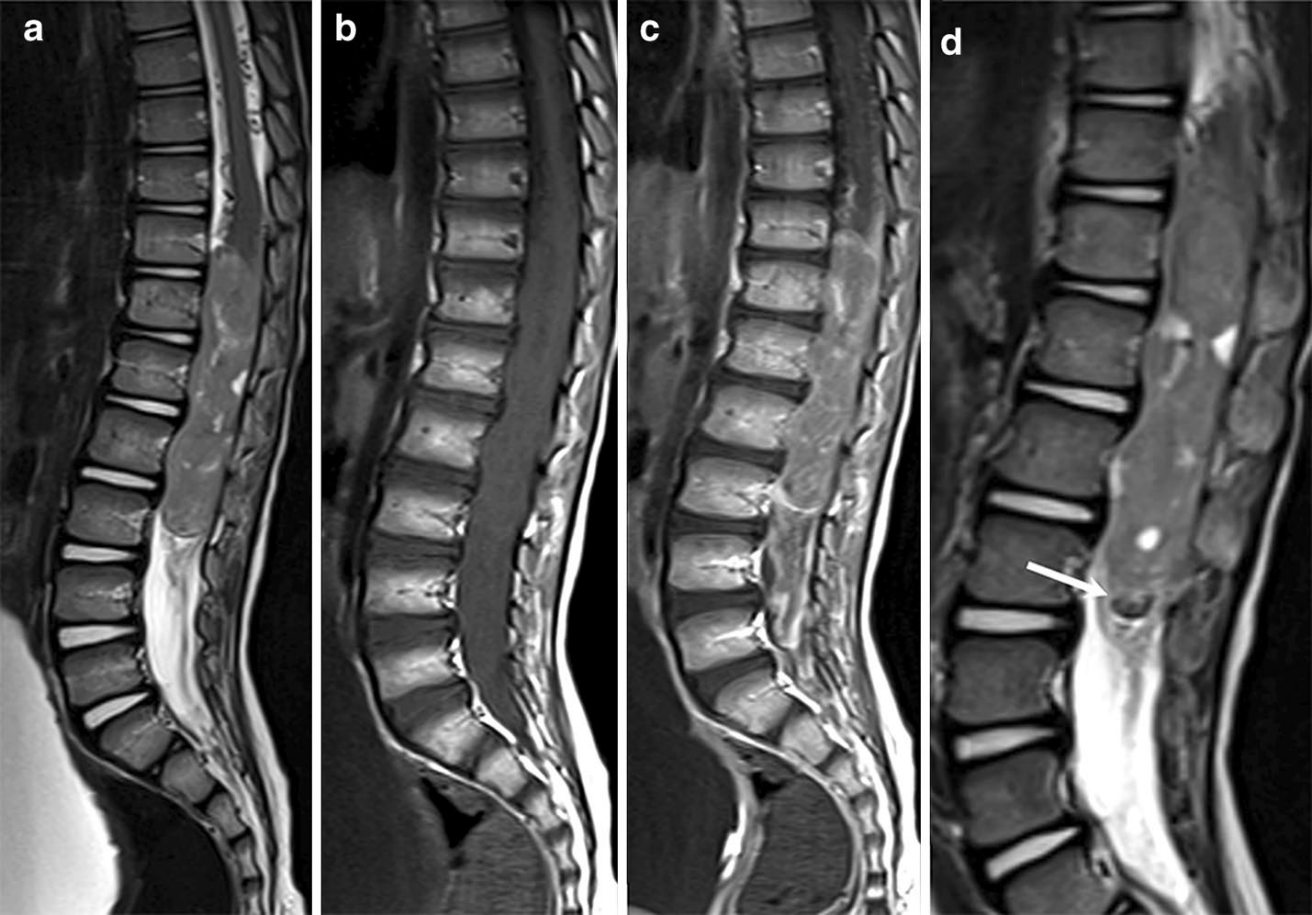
Magnetic resonance imaging (MRI) of a 4-year-old boy with spinal glioblastoma multiforme (GBM) of the conus medullaris at the time of diagnosis. MRI shows a large heterogeneous mass extensively filling the spinal canal between T11 and L3. The lesion shows hyperintense and inhomogeneous signal intensity on Sagittal T2-weighted images (a) and isointense signal intensity on Sagittal T1-weighted images (b). After gadolinium (Gd) injection, a diffuse, inhomogeneous enhancement of the tumor is observed, and the tecal sac is filled by abundant enhancing tissue enveloping the conus medullaris and cauda equina (c). The regions of hypointensity within the tumor and along the inferior margin of the lesion shown on sagittal T2-weighted images suggest tumoral bleeding (*arrow*) (d)
